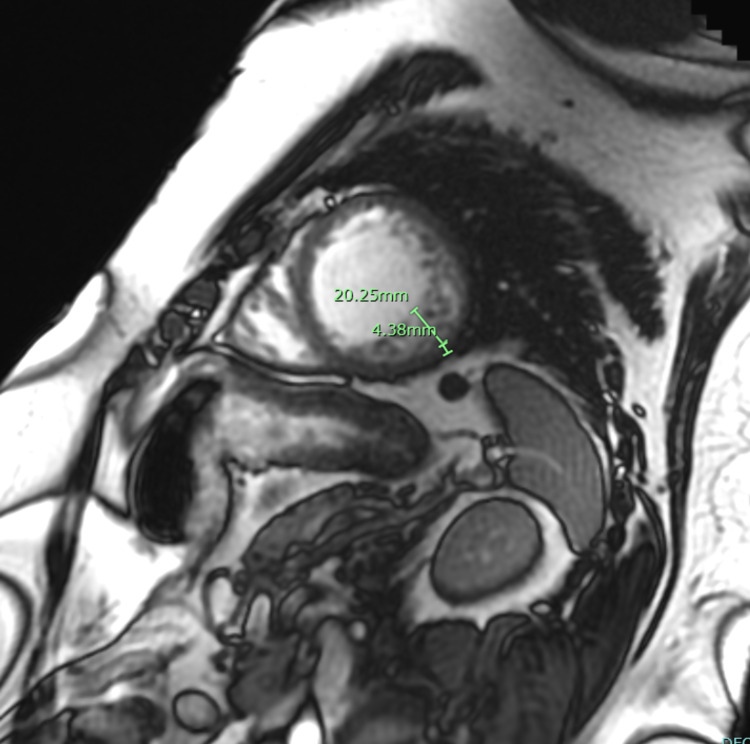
Cardiac MRI revealed in the inferolateral area, mid left ventricle the non-contrast fraction measuring approximately 20mm and the underlying compacted left ventricular wall measures 4mm. There is apical thinning and highly trabeculated.
